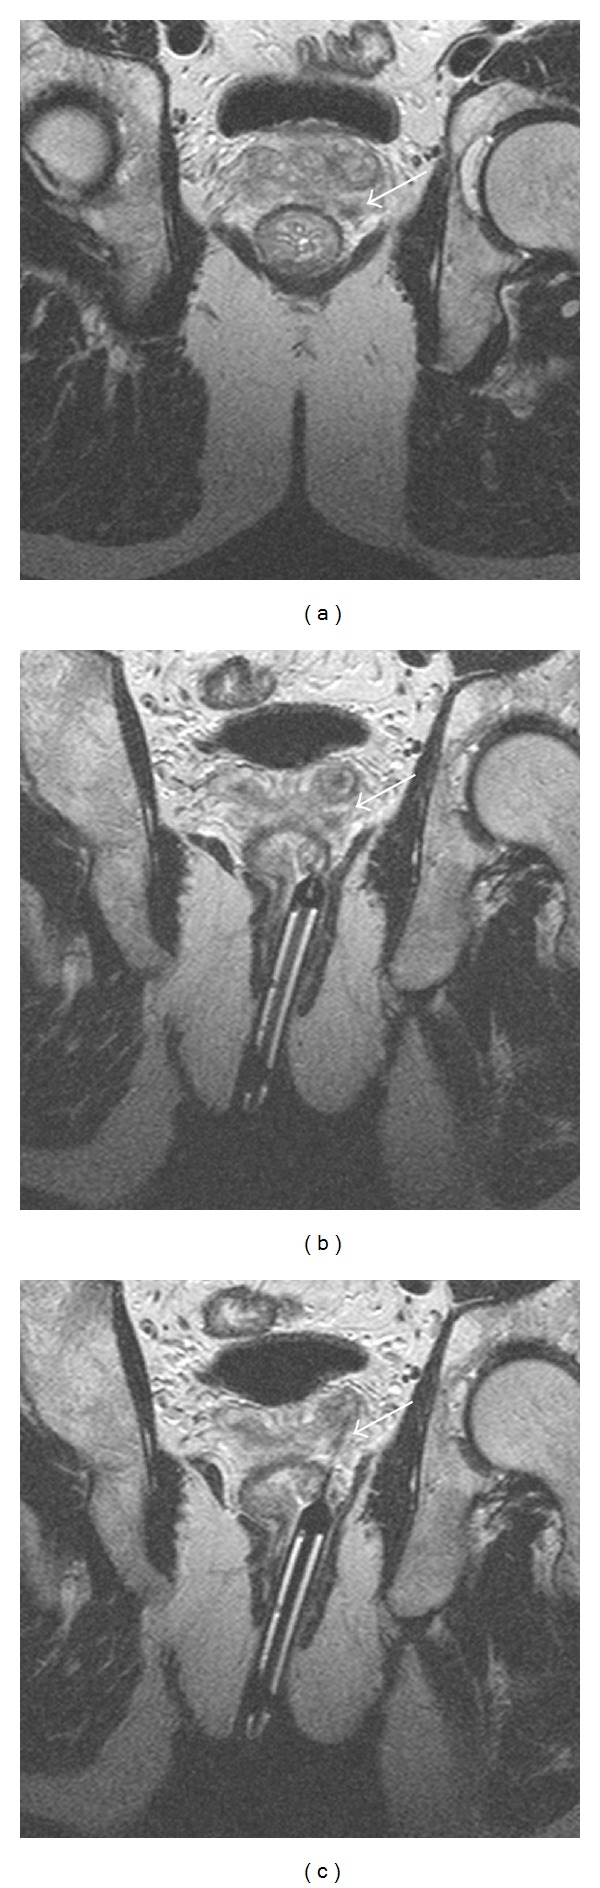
Prostate biopsy in a 1.5-T MRI system. (a) MR-image of a prostate gland with tumor-suspected area. (b) Prostate gland with needle guidance being positioned towards the tumor-suspected area. (c) Biopsy needle in the tumor-suspected area. In every image, the tumor-suspected area is marked by an arrow.
